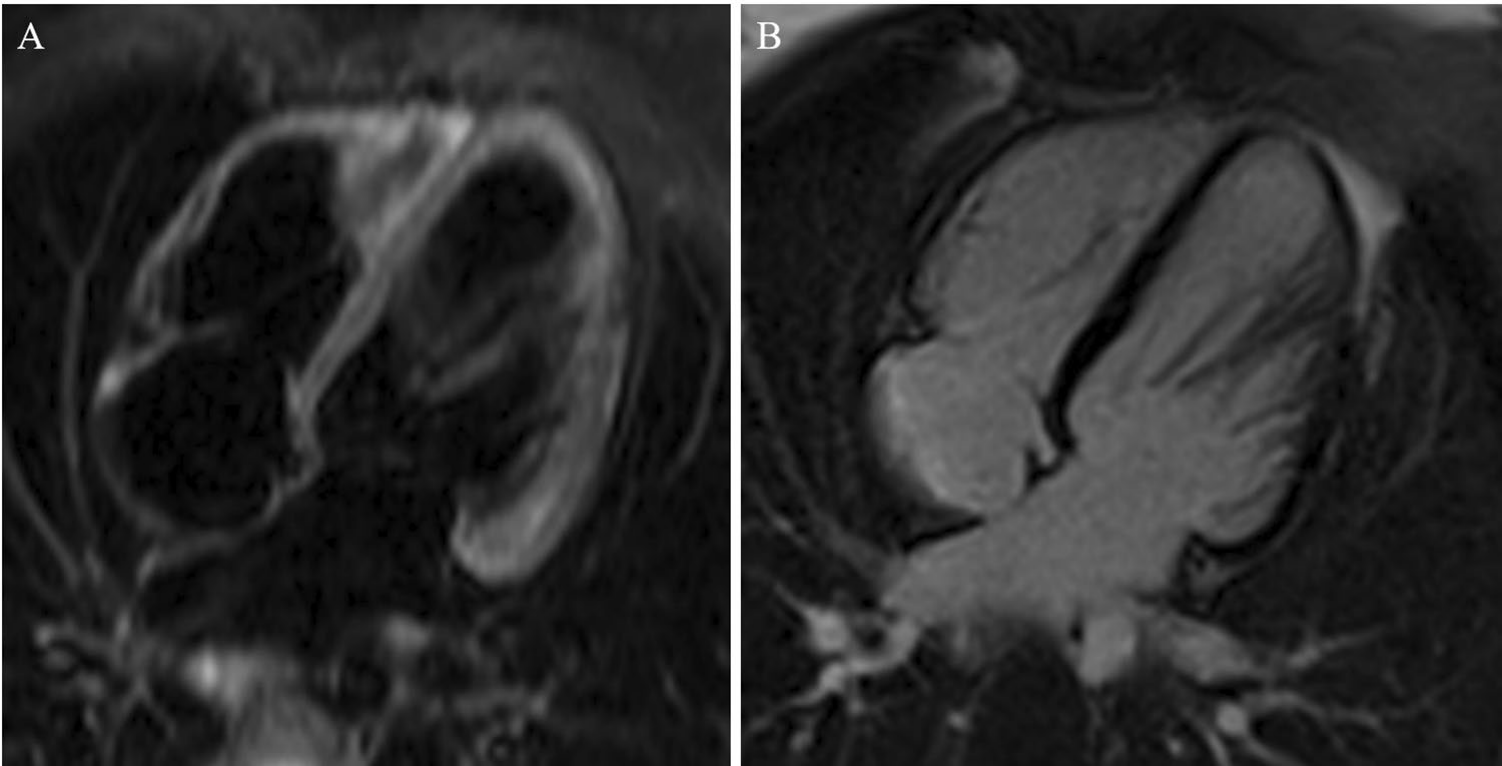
Cardiac MRI (4-chamber-view exemplarily) showing A no myocardial edema (T2-weighted edema images), and B no residual (T1-weighted) late gadolinium enhancement
